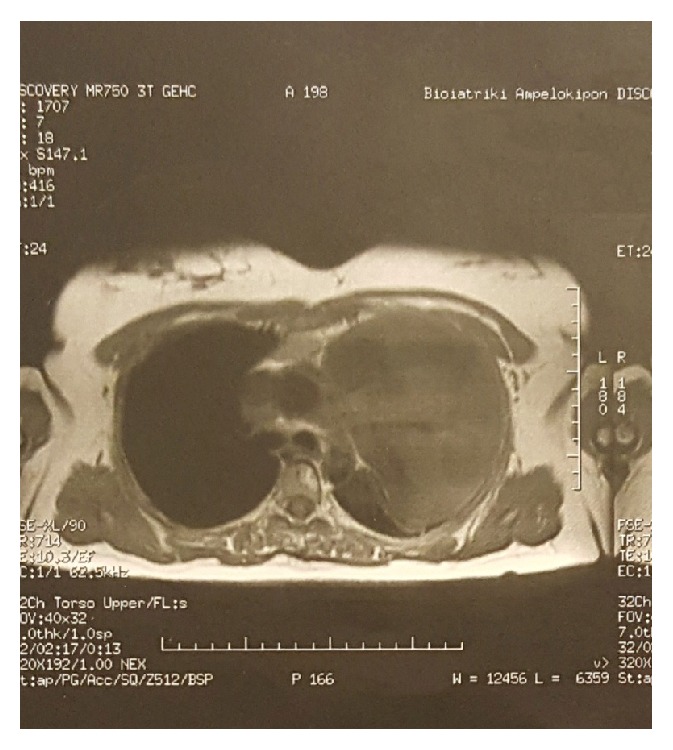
MRI of the intrathoracic cystic lesion. Osteolytic lesion in the thoracic vertebrae also obvious.
